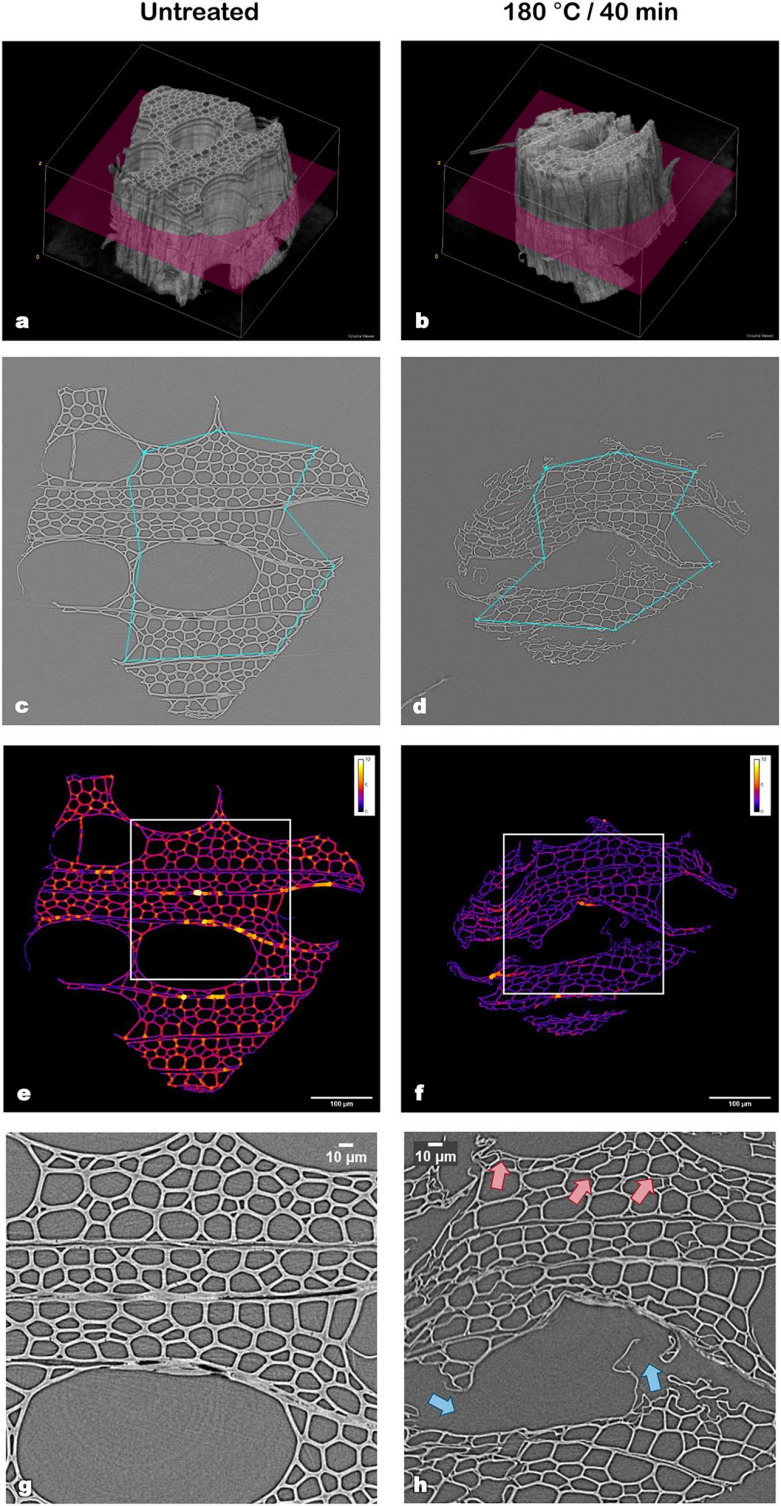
X-ray nano-tomography images of the same sample in its untreated state and after hydrothermal treatment at 180 °C for 40 min. (a,b) 3-D reconstructions of the sample, highlighting the virtual sections observed in figures (c–h) [obtained with the help of ImageJ v1.52i software; imagej.nih.gov/ij/]. (c,d) The chains of points used to determine the shrinkage of the sample. (e,f) The calculated thickness of cell walls throughout the slices (in µm) [obtained with the help of ImageJ v1.52i software; imagej.nih.gov/ij/]. (g,h) Highlights of vessel disruption (blue) and cell detachment (red), extracted from the regions within the white rectangles in (e,f).
